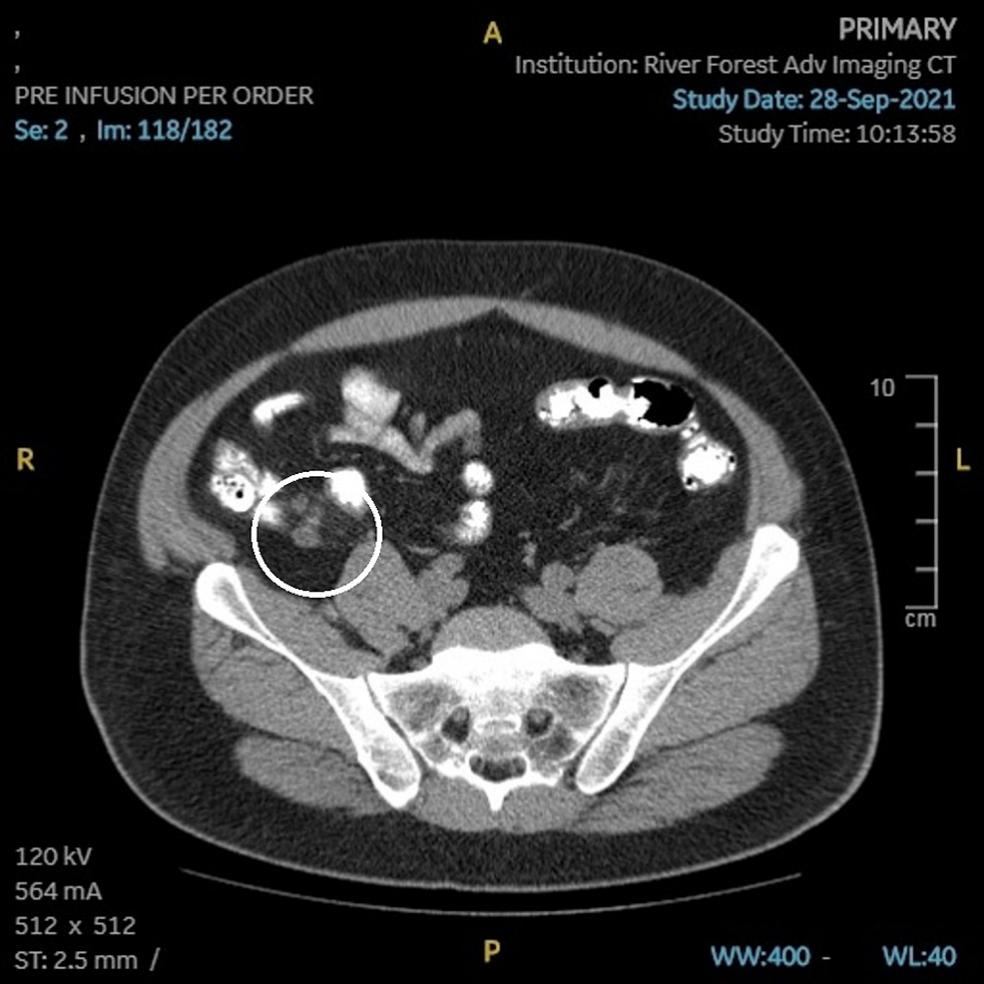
CT (transverse) image showing enlargement of the appendix and cluster of lymph nodes (circled).
